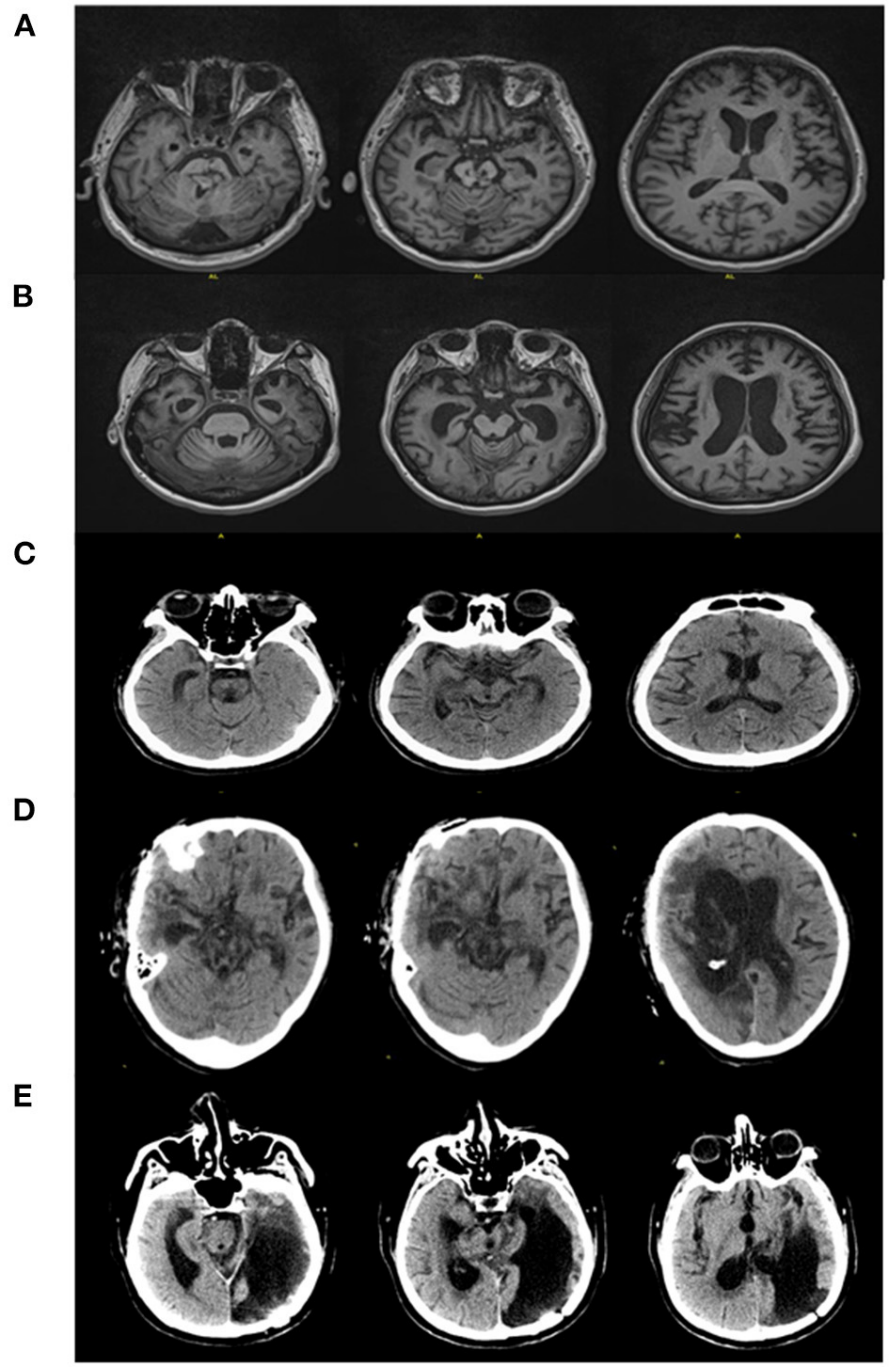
CT/MRI findings of patients. (A) MRI demonstrated brainstem malacia, brain atrophy (Patient 1). (B) MRI demonstrated whole-brain atrophy (Patient 2). (C) CT showed diffuse axonal injury, brainstem malacia (Patient 3). (D) CT showed postoperative changes of severe TBI, diffuse axonal injury, and brain stem malacia (Patient 4). (E) CT showed postoperative changes of severe TBI, brain stem malacia, and malacia of left occipital lobe and temporal lobe (Patient 5).
